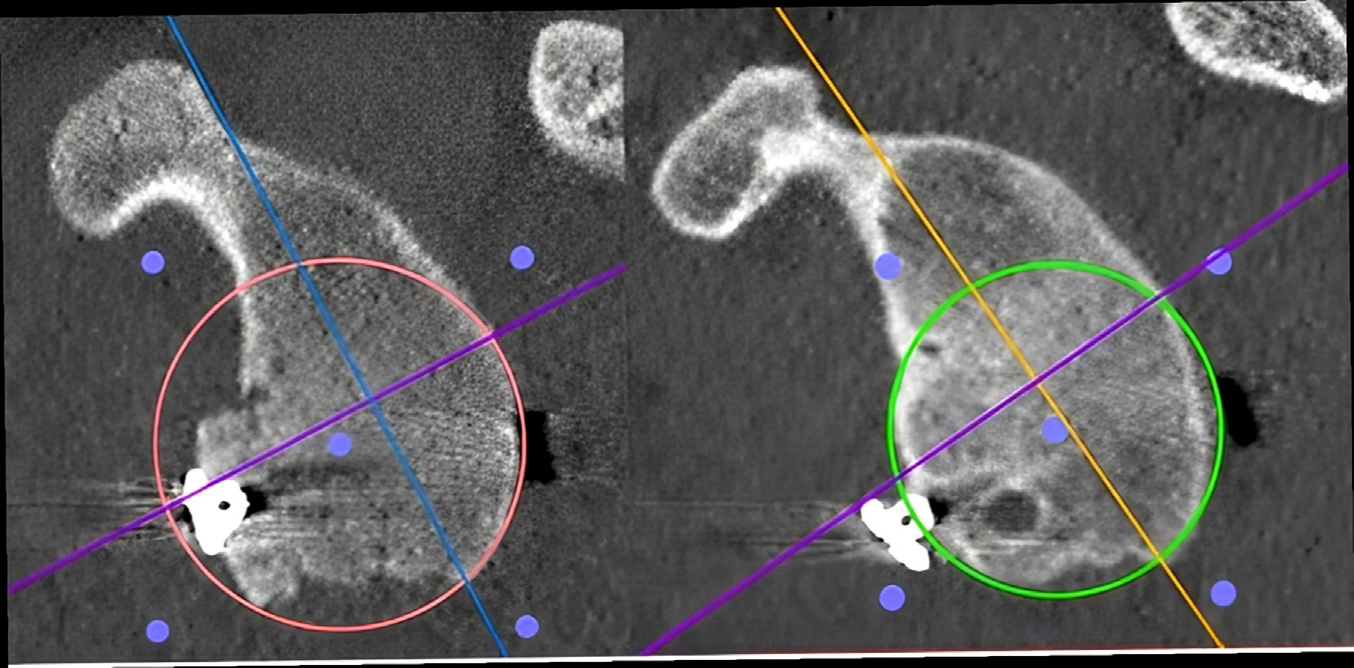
15 days postoperative (*left*) and last follow-up (*right*) CT scan evaluation. Glenoid en-face view showing the best-fit circle technique for bone reconstruction evaluation. *CT*, computed tomography.
